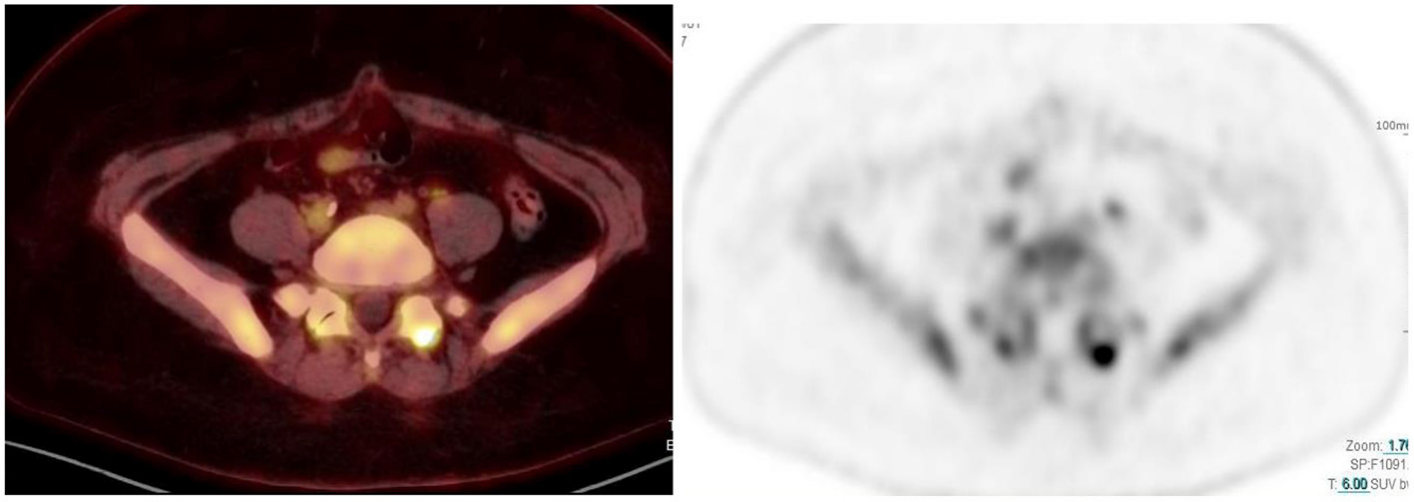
59 year old woman with breast Ca. Combined PET/CT and PET images show intense uptake in the left facet joint of L5/S1, which is osteodegenerative.
